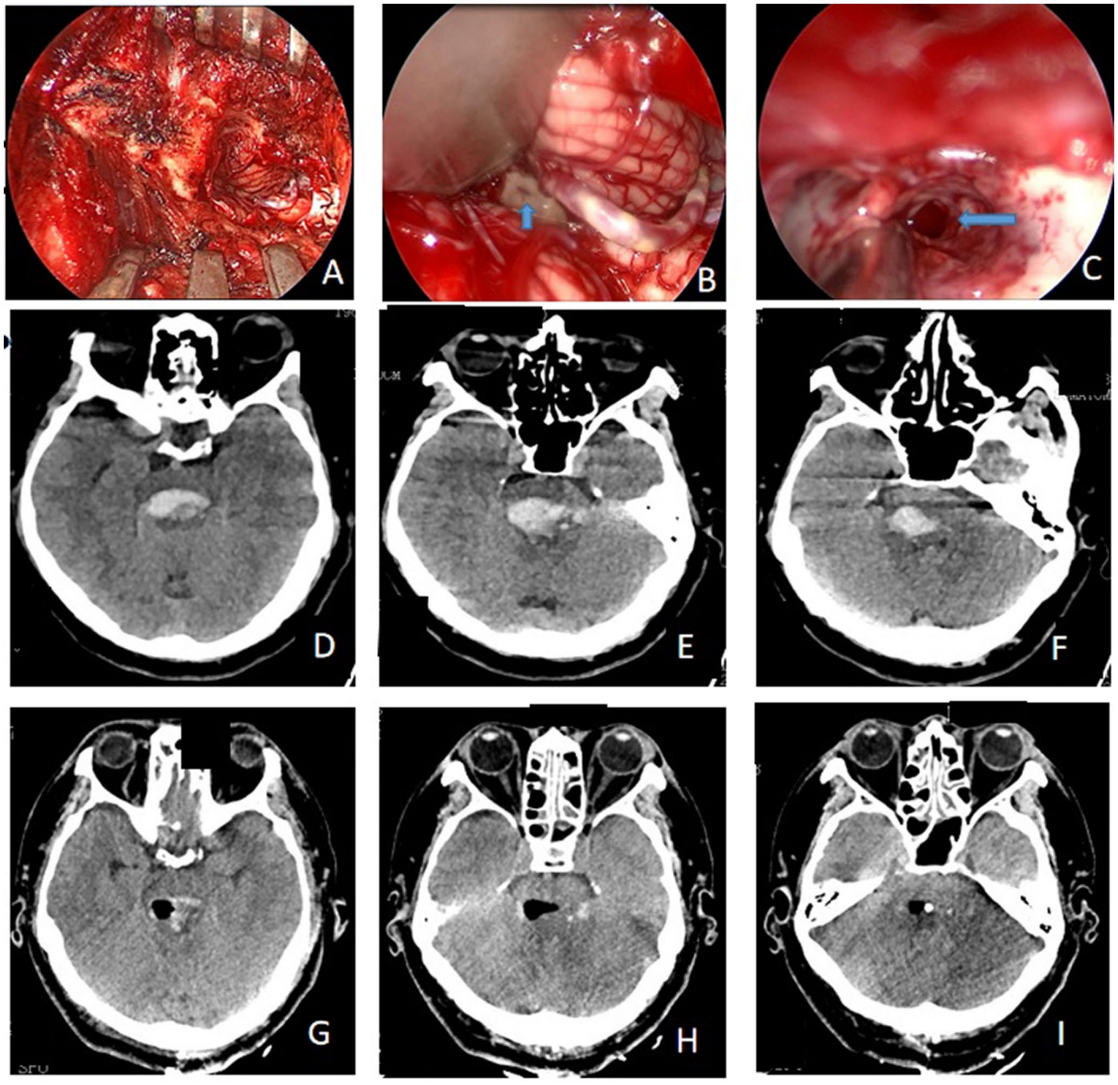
(A) Intraoperative incision and bone window; (B) the location of the hematoma was found during surgery (blue arrow); (C) the hematoma cavity after the removal of the hematoma by neuroendoscopy (blue arrow); (D–F) preoperative CT showed evidence of brainstem hemorrhage; (G–I) complete removal of hemorrhage was achieved, as confirmed by the post-op CT scan image.
